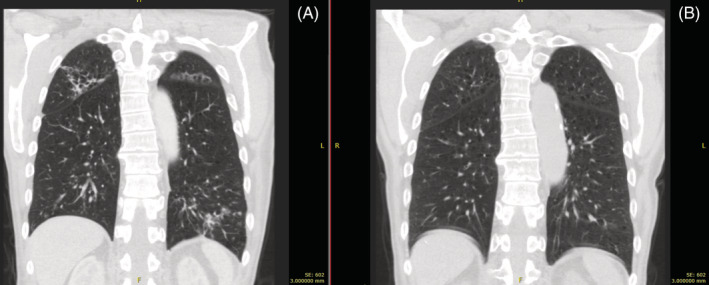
(A) Coronal CT slice of multifocal pneumonia and (B) resolution
